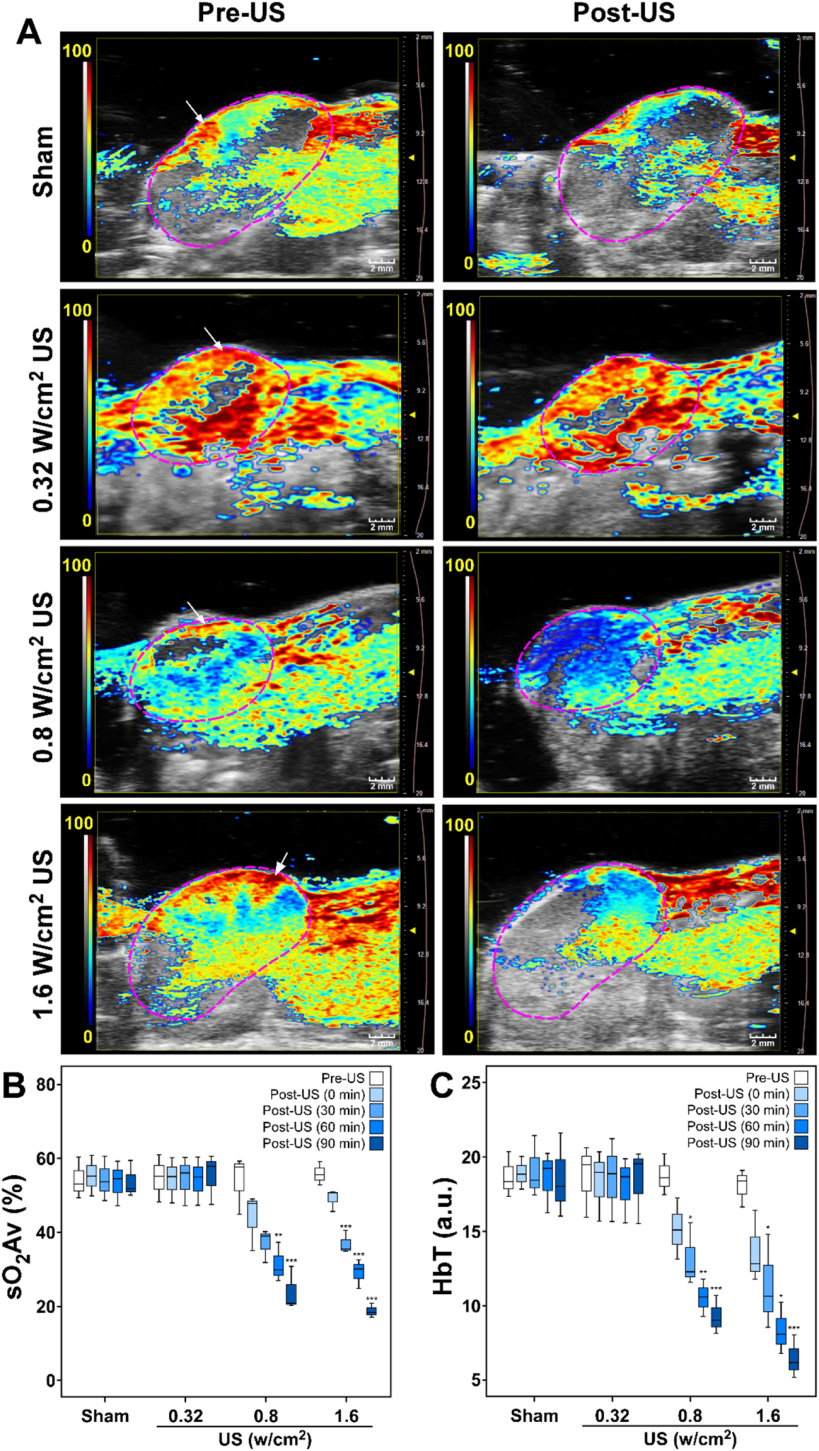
Photoacoustic assessment of HCC tumor oxygenation before and after US treatment. Three different US treatment groups with I_SATA_ of 0.32 W/cm^2^ (peak pressure = 0.23 MPa), 0.8 W/cm^2^ (peak pressure = 0.36 MPa), and 1.6 W/cm^2^ (peak pressure = 0.23 MPa) were investigated and compared. (A) Representative two-dimensional PA images of tumors displaying blood oxygen saturation maps with co-registered grayscale B-mode images acquired before (Pre-US) and 90 min after (Post-US) ultrasound therapy. Oxygenation level ranges from 0% (dark blue) to the 100% (dark red). White arrows show high PA signal in the periphery of the tumor. The boxplots display the minimum, first quartile, median, third quartile, and the maximum of the oxygenation measurements for (B) sO_2_ average (sO_2_Av) and (C) total hemoglobin (HbT) in the whole tumor volume. Mean values of sO_2_Av or HbT in the whole tumor volume for the sham, 0.32, 0.8, and 1.6 W/cm^2^ US treatment groups were calculated and compared, Pre-US versus Post-US. *, , and * represent statistical significance of *p* ≤ 0.05, *p* ≤ 0.01, and *p* ≤ 0.001, respectively. Scale bar 2 mm.
